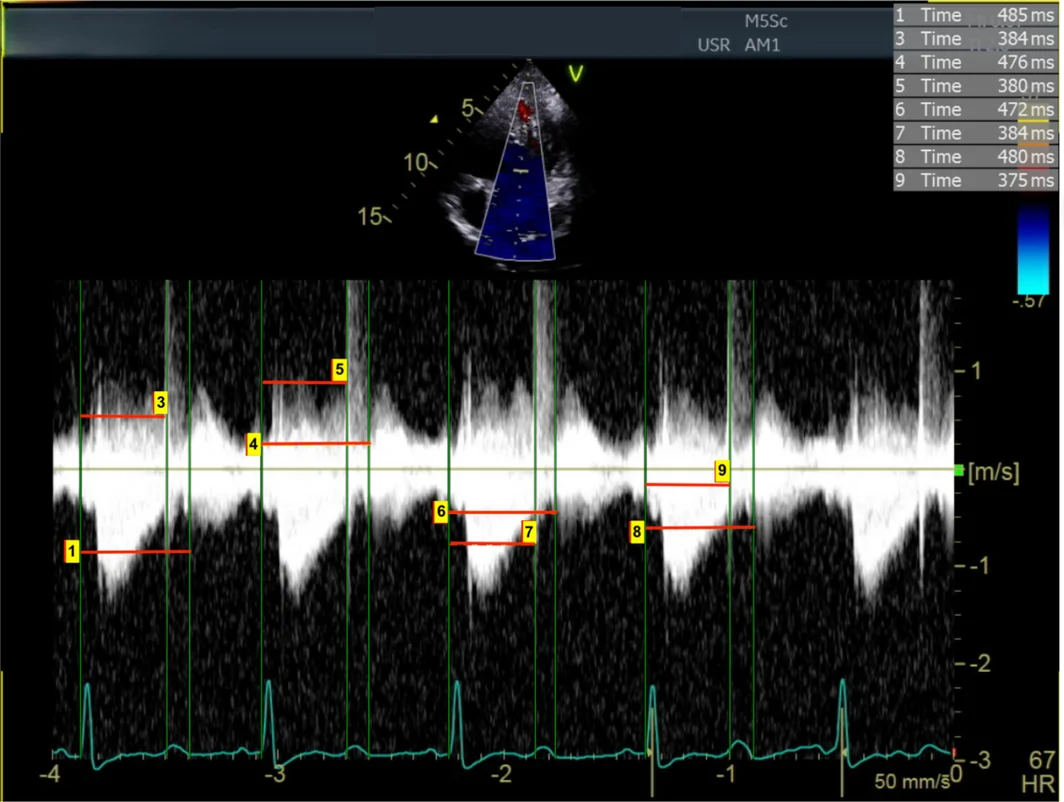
Representative measurement of the electromechanical window (EMW). The green calipers represent timing measurements generated by the echocardiographic software. Measurement 1 corresponds to the QT interval (Q onset to end of T wave; 485 ms), while Measurement 3 corresponds to mechanical systole (Q onset to aortic valve closure; 384 ms). EMW was calculated as mechanical systole minus QT interval (384–485 = −101 ms). Measurements were repeated across multiple cardiac cycles, yielding a mean EMW of −97.5 ms.
